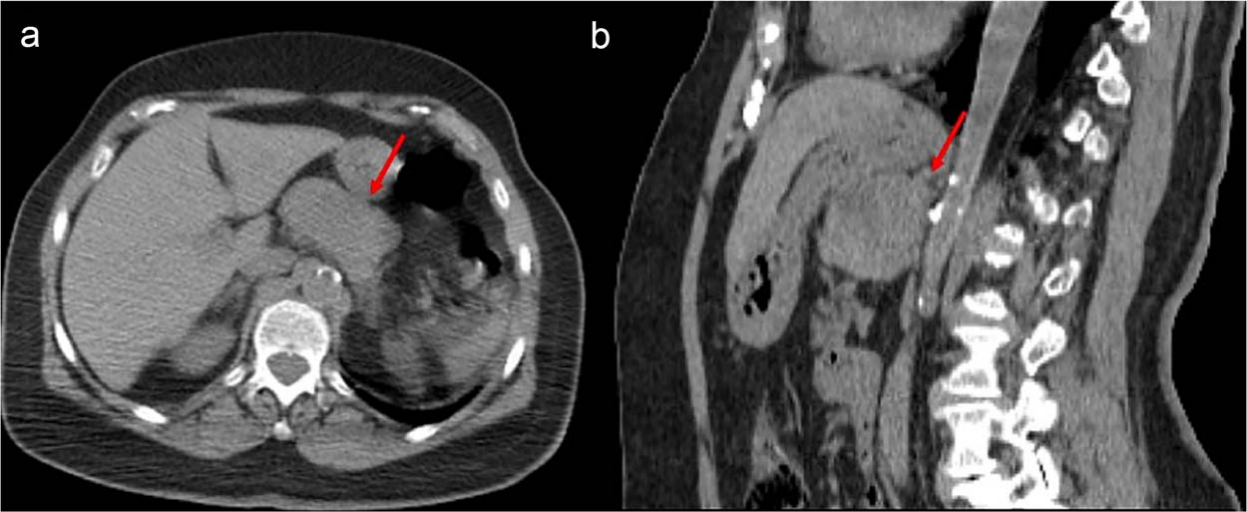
Unenhanced CT scan, axial (a), and sagittal reconstruction (b), revealing a well-defined oval mass with regular contours and soft-tissue density, closely contacting the posterior wall of the stomach and appearing to occupy the omental bursa. The lesion does not invade adjacent structures.
